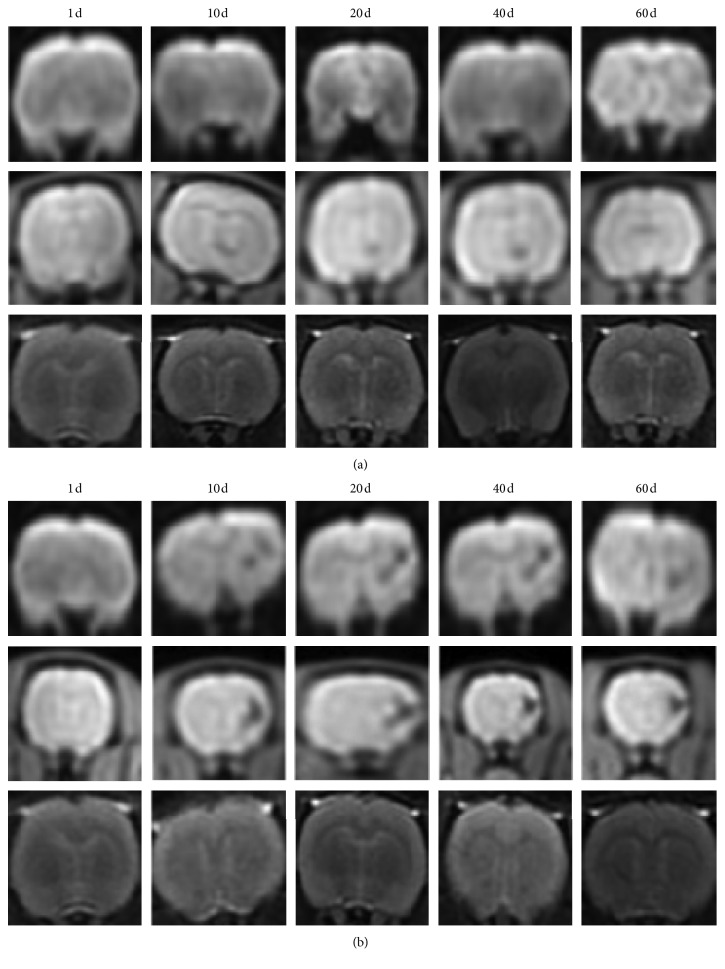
MRI images (DWI, SWI, and T2WI sequences) after transplantation (1 d, 10 d, 20 d, 40 d, and 60 d). (a) SPIO-BMSCs. As time goes on, hypointense signals could still be visible on days 10, 20, and 40 in the SWI sequence. (b) *Fth1*-BMSCs. Hypointense signals could still be visible on days 10, 20, 40, and 60 in the SWI sequence.
